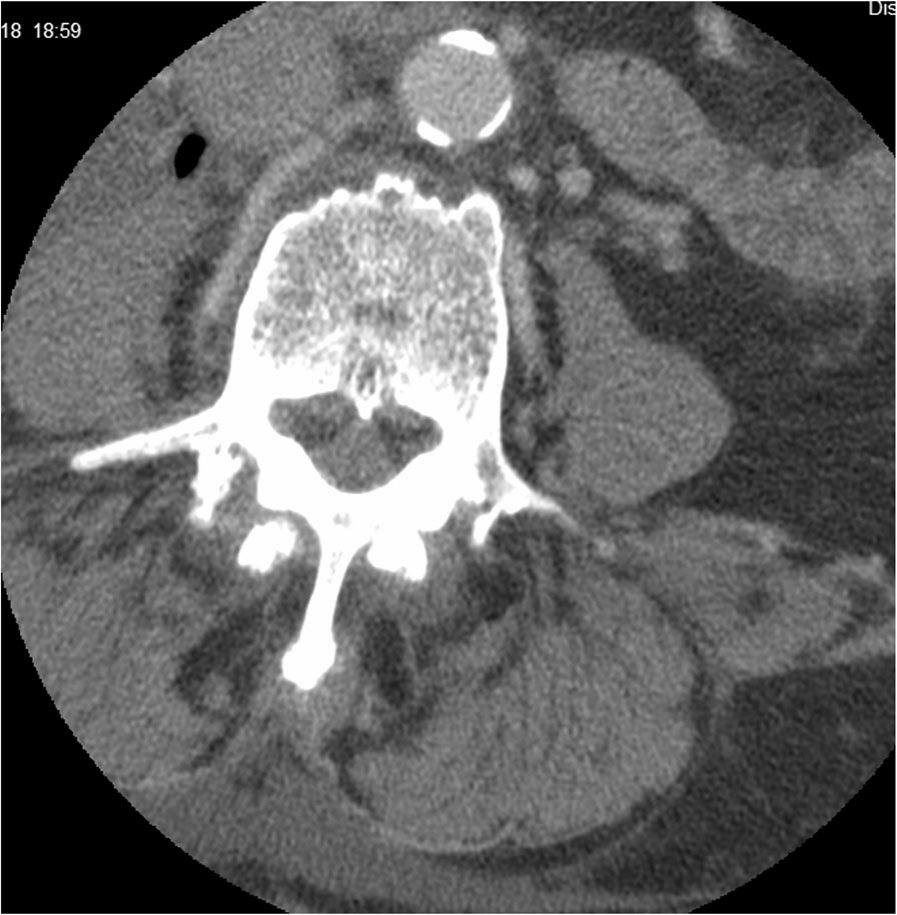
Axial CT lumbar spine: note air within the abscess in the right iliopsoas muscle
